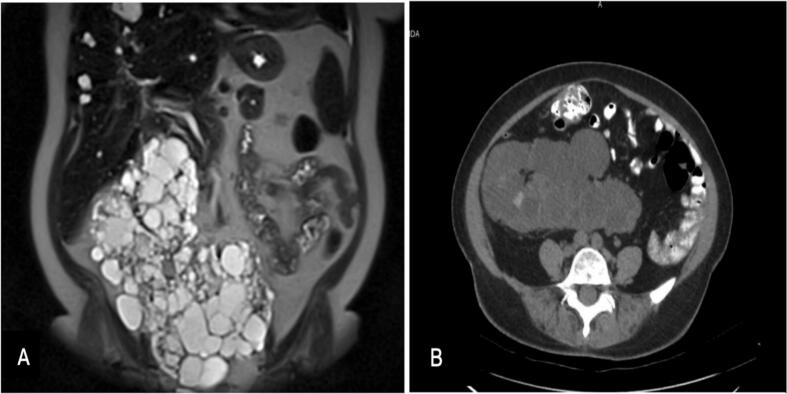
(A) MRI Abdomen with IV Contrast enhancement T2 weighted image (Coronal view) demonstrates a large, lobulated, multi-cystic structure in the midline, consistent with a horseshoe kidney exhibiting polycystic changes. The enlarged cystic kidneys seem to displace the surrounding structures, including the bowel and abdominal organs. (B) CT Scan of the Abdomen/Pelvis with PO and IV Contrast enhancement (Axial view) demonstrates hypodense cysts inside the horseshoe kidney.
